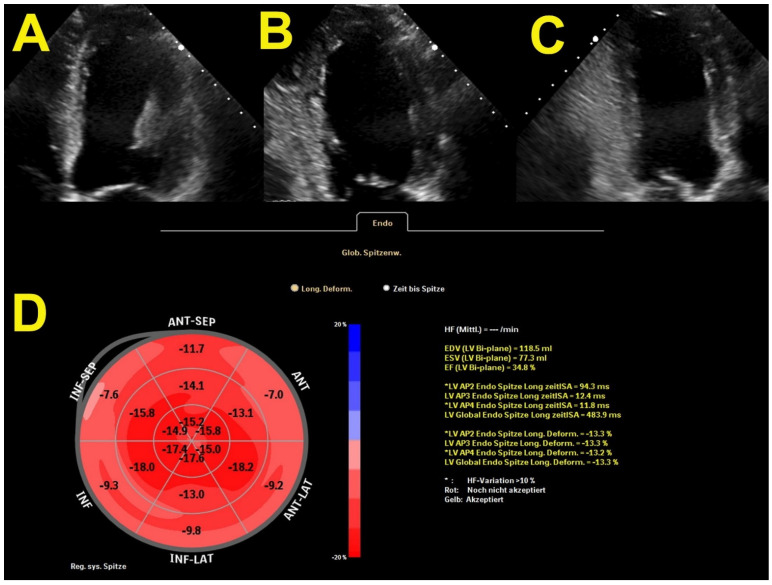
Echocardiographic assessment of left ventricular longitudinal strain with bullseye presentation. (A) 4-chamber view, (B) 2-chamber view, (C) 3-chamber view, (D) bullseye presentation of longitudinal strain of each myocardial segment. EDV—endi-diastolic volume, ESV—end-systolic volume, EF—ejection fraction, LV—left ventricicle, AP2—two-hamber view, AP3—three-chamber view, AP4—four-chamber view, ANT-anterior, SEP-septum, LAT-lateral, INF-inferior.
